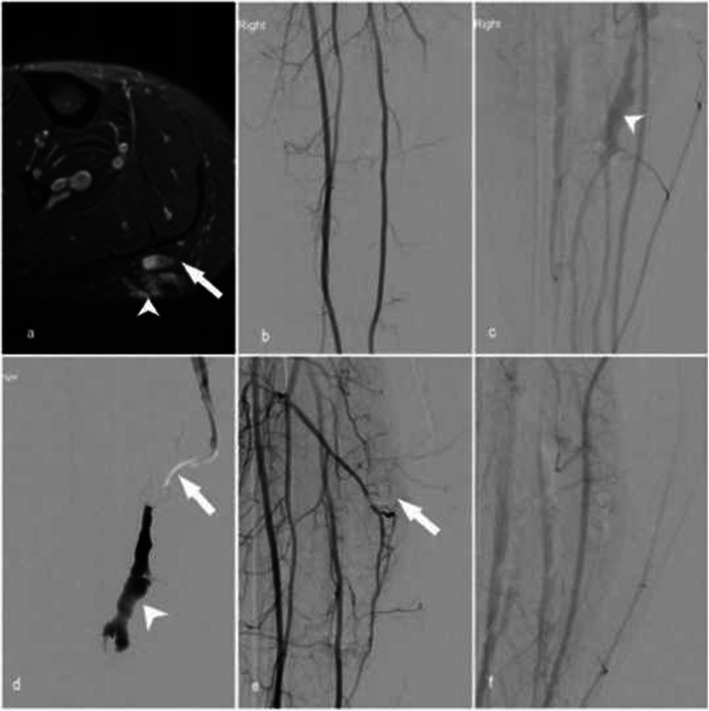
Patient 1. a) Axial post contrast T2 weighted fat saturated MRI demonstrating a lobulated slow-flow vascular malformation in the posterior calf measuring 5.7 cm with fluid haematocrit levels (arrowhead) with dilated draining veins (arrow). b) Digital subtraction angiography did not reveal arterial inflow compatible with a slow-flow venous malformation. c) Venous phase of the angiogram showing venous pouch d) Digital subtraction venography showing retrograde venous access with 6Fr intermediate catheter (Sofia, Microvention, etc) (arrow) placed within the venous pouch (arrowhead). e) Arterial inflow was then controlled using a pressure cooker technique with inflation of a dual balloon catheter (Scepter C, Microvention, etc) (arrow). 6 ml sodium tetradecyl sulphate 3% was injected from the venous side with f) subsequent arteriogram confirming no evidence of arterial leak or distal embolization and successful occlusion of the venous malformation
